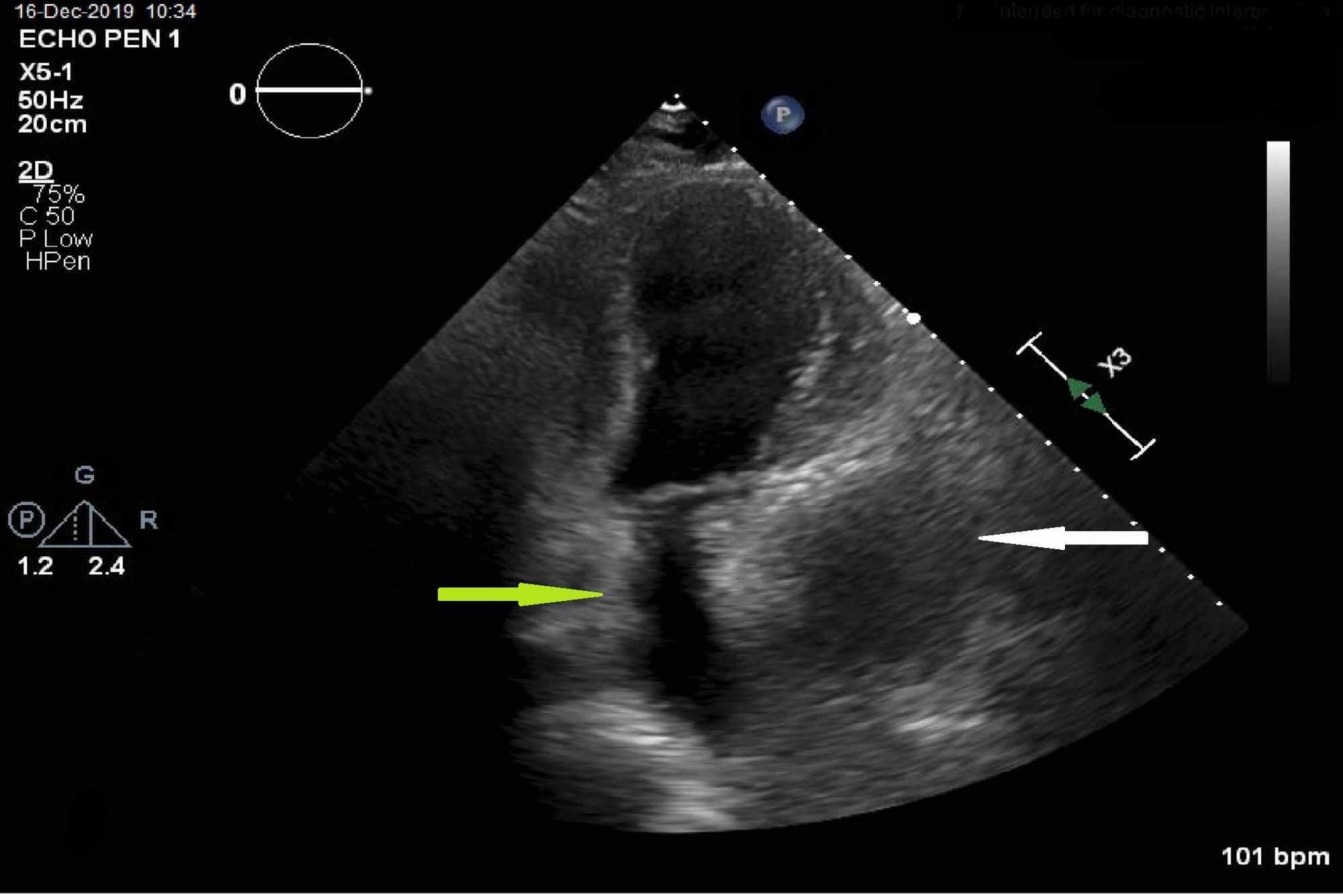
Transthoracic echocardiogram Transthoracic echocardiogram, apical two-chamber view, shows an extracardiac structure (white arrow) compressing the left atrium (green arrow) and mitral annulus.
